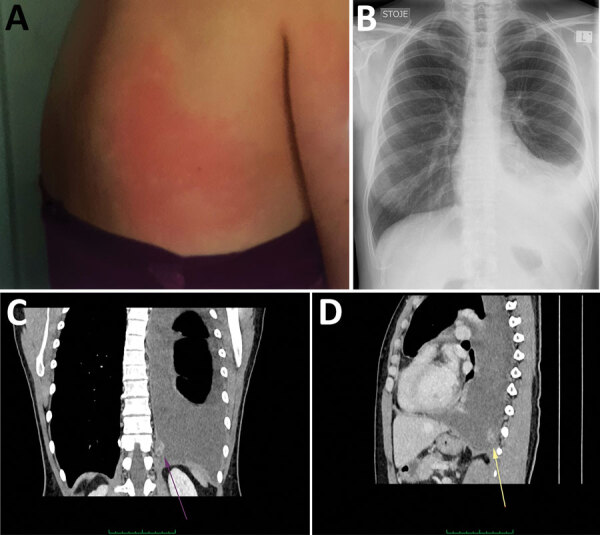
Dirofilaria repens* infection with subcutaneous and pleural manifestations in a woman in Slovenia. A) Erythematous itchy skin lesion on the patient’s back measuring 15 cm x 15 cm (photograph taken by the patient). B) Frontal radiograph showing large left-sided pleural effusion. C, D) Contrast-enhanced computed tomography images showing large left-sided pleural effusion, uneven thickening of pleura, and a focal, heterogeneously enhancing soft tissue mass measuring 26 mm × 16 mm × 14 mm (arrows) in the posterior inferior part of the costal pleura in the coronal (C) and sagittal (D) plane.
